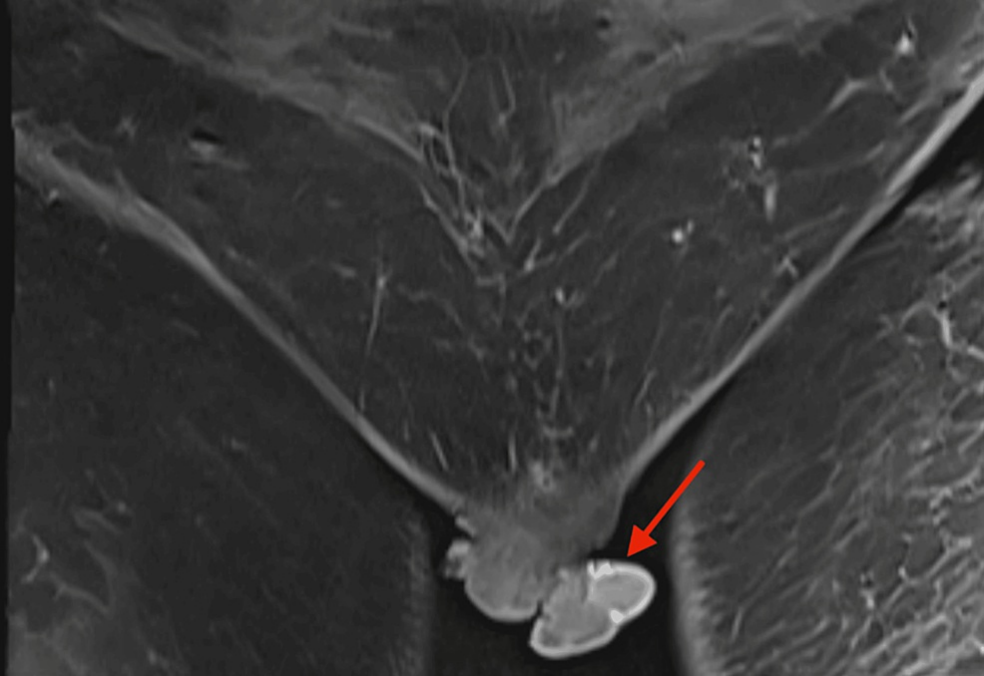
Coronal section of T1-weighted MRI of pelvis The image shows a large solid irregular mass with a polypoidal outline in the vulva involving both labia majora with exophytic extension MRI: magnetic resonance imaging
